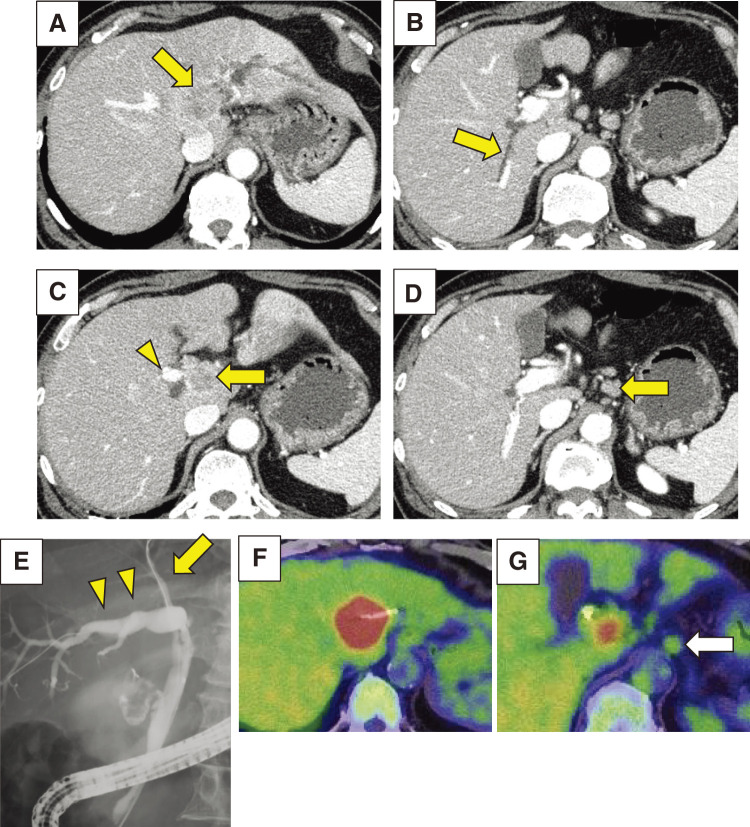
Findings before chemotherapy. (A) Dynamic enhanced CT at initial assessment showed a perihilar tumor (arrow), 27 mm in diameter, with an upstream dilatation of the intrahepatic bile ducts. (B) Posterior bile duct was dilatated (arrow) in the same enhanced CT. (C) Anterior branch of portal vein (arrowhead) was adjacent to the tumor (arrow). (D) Bulky lymphadenopathy (arrow) was detected by the same enhanced CT. (E) ERCP showed obstruction of left hepatic duct, dilatated right posterior bile ducts (arrowhead), and the modest dilatation in anterior bile duct (arrow). (F) ^18^F-FDG PET-CT showed strong uptake at the intrahepatic tumor. (G) Lower uptake was observed at the lymphadenopathy in the same ^18^F-FDG PET-CT (arrow). CT, computed tomography; ERCP, endoscopic retrograde cholangiopancreatography; ^18^F-FDG PET-CT, fluorin-18 fluorodeoxyglucose positron emission tomography–computed tomography
